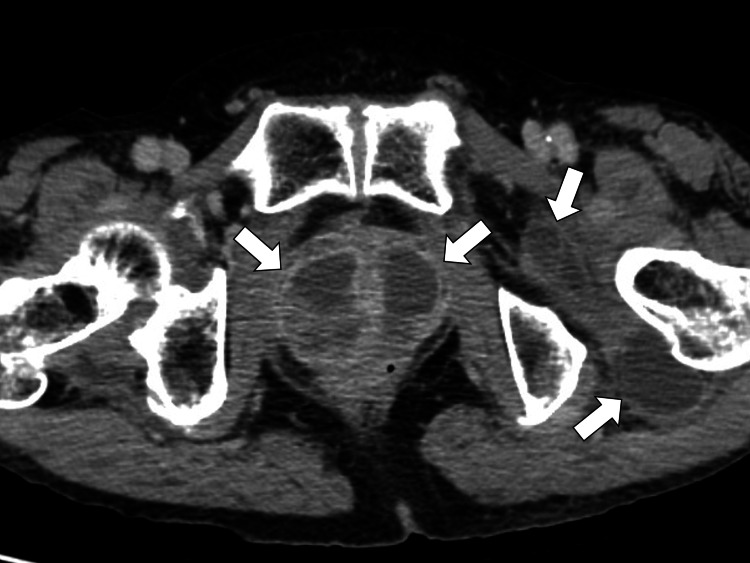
Initial contrast-enhanced computed tomography showing multiple abscess formation in the prostate and perifemoral spaces (white arrows)
